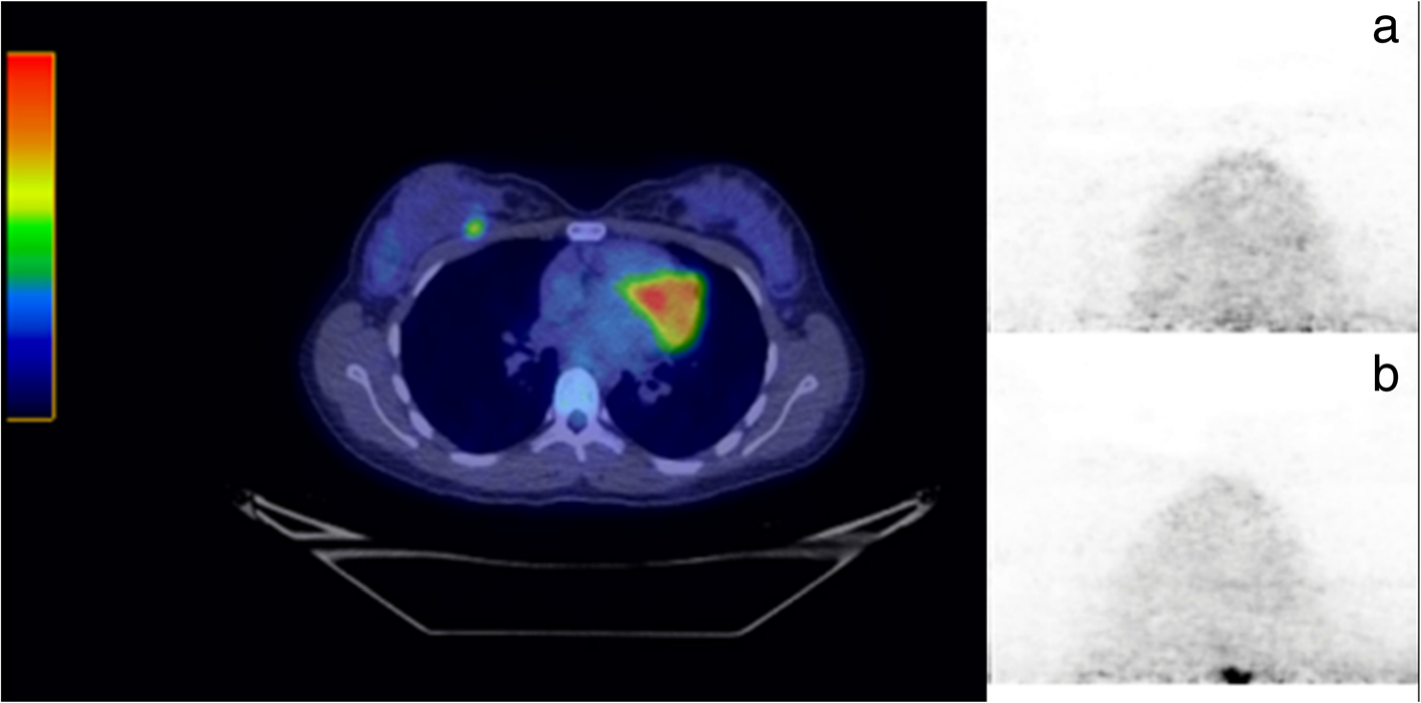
PET/CT image of a patient with metastatic lesion from malignant melanoma, located between the ribs and breast. A sub-centimeter focus of increased FDG avidity is seen within the deep soft tissues of the medial right breast. The lesion was not visible on the db-PET image acquired with the standard aperture (a), but can just be visualized at the posterior edge of the images acquired with the medium (200 mm) aperture, with an estimated size of 11.5 mm (b)
